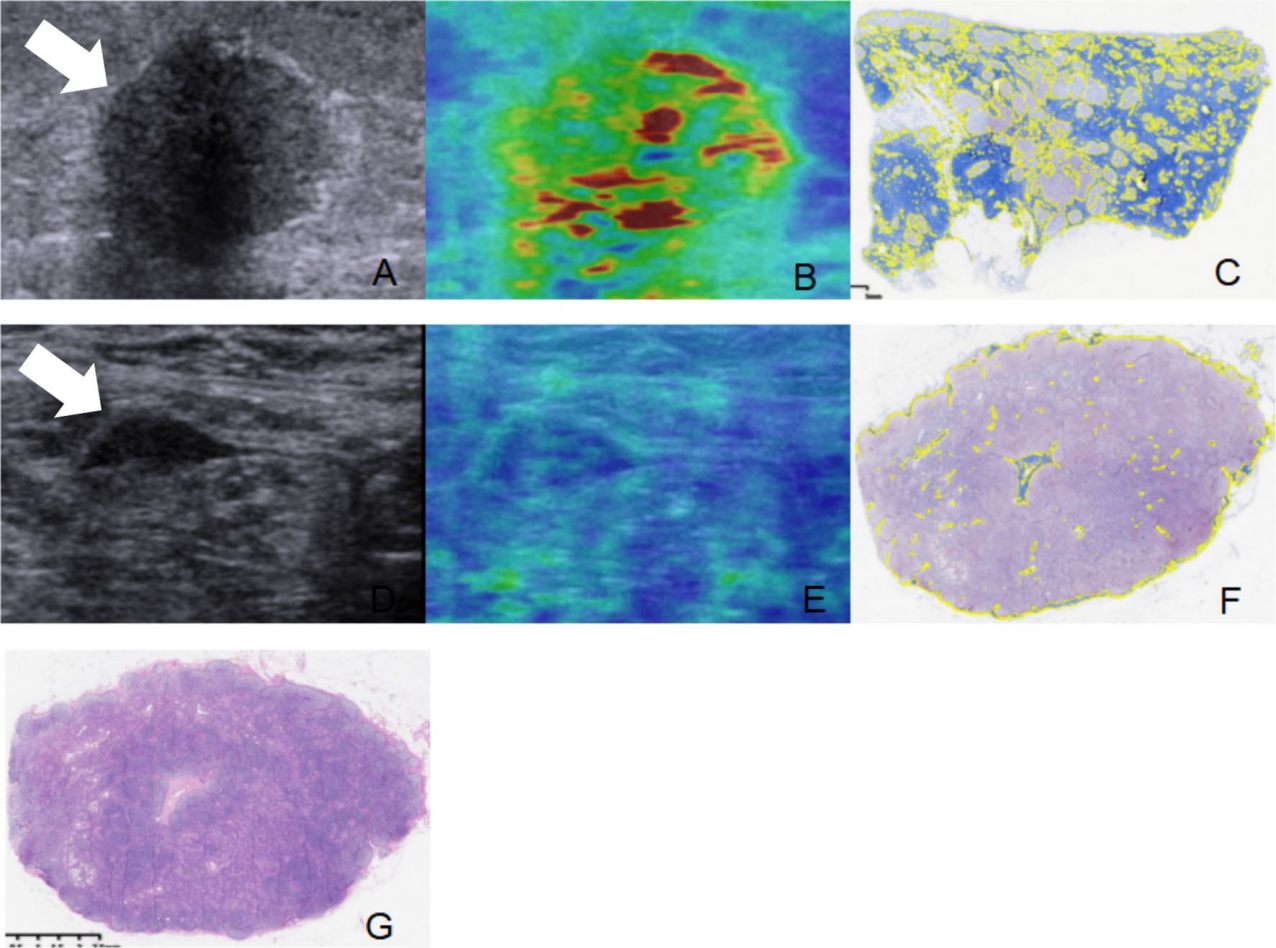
A breast cancer case with residual metastasis in axillary LNs after NAC. The patient was a 36 year-old female with Luminal B breast cancer who received eight courses of NAC. After the completion of NAC, a residual breast lesion (arrow) with a size of 18 × 13 mm was observed (A). The SWV of the breast lesion was measured to be 5.36 m/s (B), which was higher than threshold value of 2.47 m/s. In addition, the CVF of the breast lesion was found to be 62.88% (C), exceeding the threshold value of 37.67%. D shows a LN (arrow) with suspicious features on US. This LN exhibited a size of 26 × 8 mm, an outwards bulging shape, and a focal cortical thickening of 4.4 mm. The SWV of the LN was measured to be 1.48 m/s (E), and the CVF was 4.5% (F). Following NAC, a total of 19 axillary LNs were resected, and metastasis was found in two of them (G). LN, lymph node; NAC, neoadjuvant chemotherapy; SWV, shear wave velocity; CVF, collagen volume fraction; LN, lymph node; US, ultrasound
